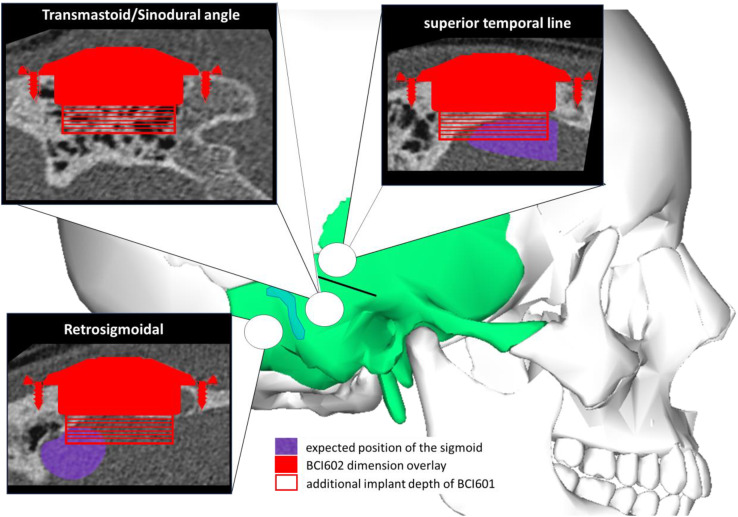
Representative case for surgical planning via OTOPLAN CT images in OTOPLAN showing placement of the BCI602 (red) and additional space required for the BCI601 (broken line) in the three positions as indicated in the picture: (a) retrosigmoidal, (b) transmastoid/sinodural angle, (c) superior to temporal line outlined in an anatomical sketch. CT images are from subject BCI602_13. Positions (a) and (b) would require usage of lifts in the particular case.
